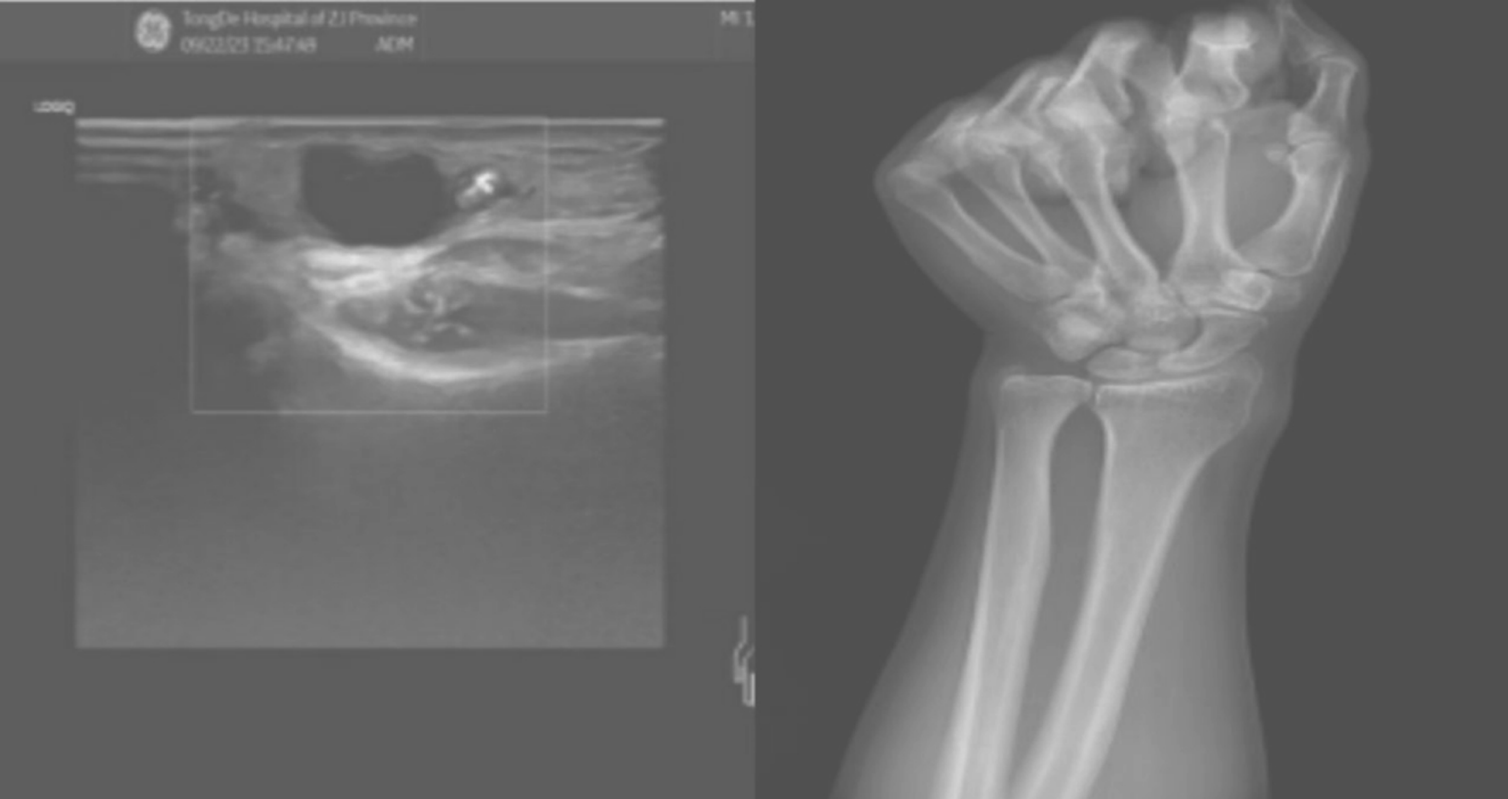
Preoperative ultrasound and X-ray.
